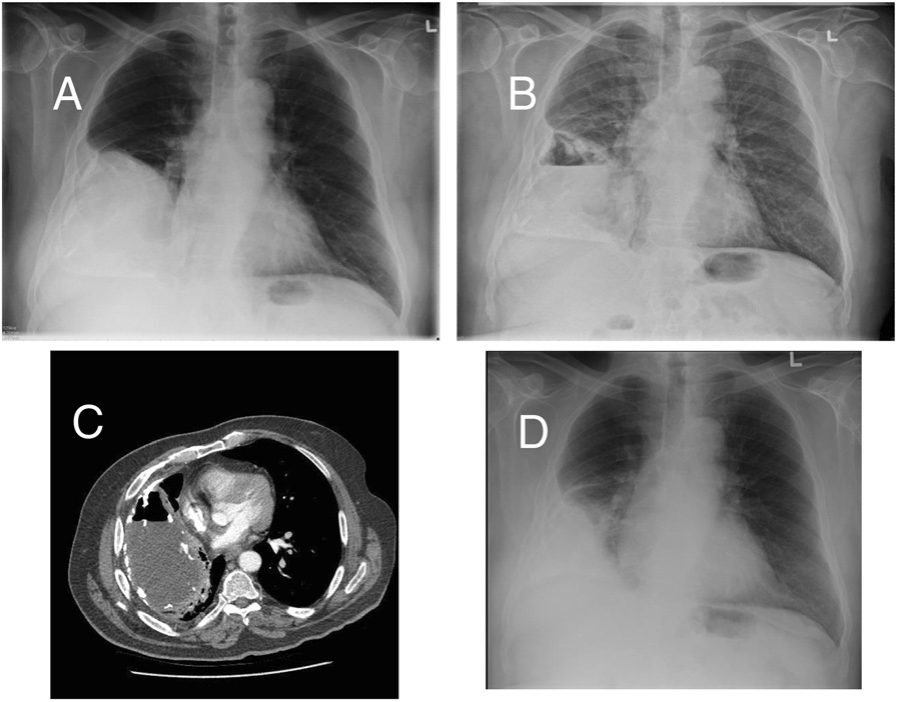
Panel a: A posterior-anterior (PA) chest radiograph dated November, 2010 showing a large loculated pleural effusion on the right side. Earlier radiographs (now purged) were reported to show a similar abnormality. Panel b: A PA chest radiograph dated January, 2012 showing an air-fluid level in the previously described loculated effusion. It is consistent with the interval development of a bronchpleural fistula. Panel c: A computed tomographic image dated June, 2012 showing right sided volume loss and a peripherally calcified loculated right hydropneumothorax. Panel d: A PA chest radiograph dated May, 2015 showing the previously described chronic loculated effusion to be reduced in size. An air-fluid level is no longer visible
